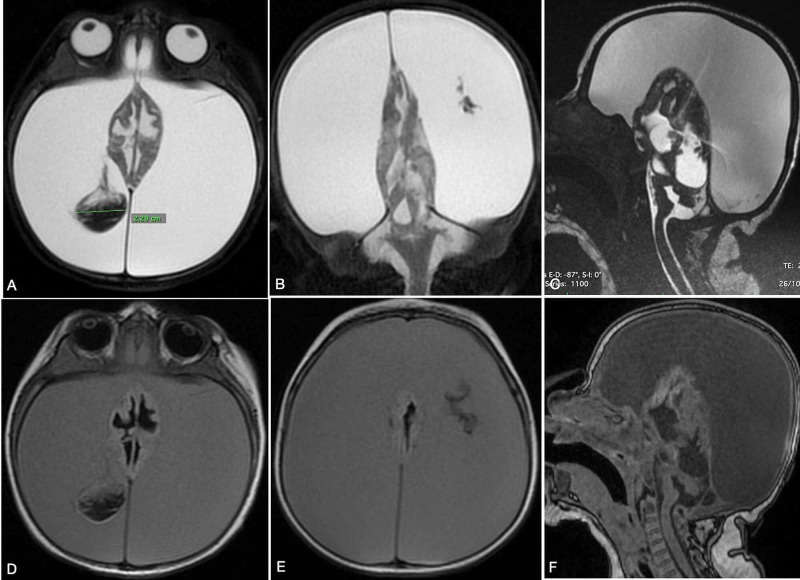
Massive subdural collection on MRI Axial T2-weighted MRI showed a peduncular hypointense mass in the collapsed brain and hyperintense subdural fluid collection (A). Coronal T2-weighted MRI showing a midline collapsed brain (B). Sagittal T2-weighted MRI showing compression of the posterior fossa content by a supratentorial increased hypertensive compartment (C). T1-weighted MRI displaying hypo-/isointense subdural fluid collection (D). Axial T1-weighted MRI high-level slice showing a massive subdural hypo-/isointense mass collapsing the brain (E). Sagittal T1-weighted MRI showed compression of the posterior fossa.
